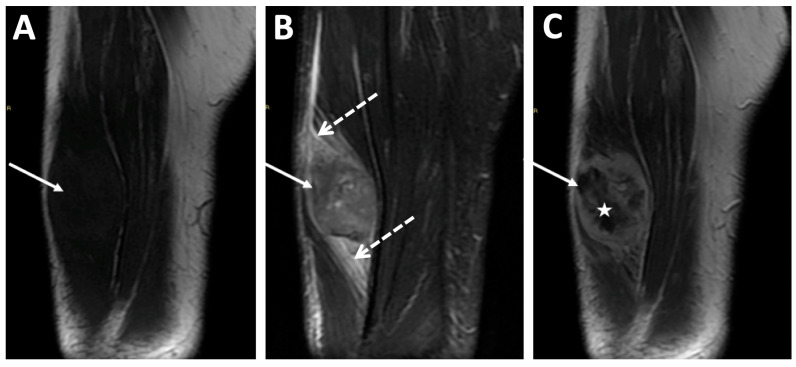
MRI of a high-grade extraskeletal osteosarcoma of the right thigh diagnosed in an 83-year-old male. (A) Coronal T1-weighted imaging (WI), (B) fat-suppressed coronal T2-WI, and (C) coronal T1-WI with gadolinium chelate injection. The tumor was seated deep in the anterior compartment of the thigh (arrows) and demonstrated a peritumoral edema (dashed arrows above and below the tumor) and inhomogeneous enhancement with internal necrosis (star).
